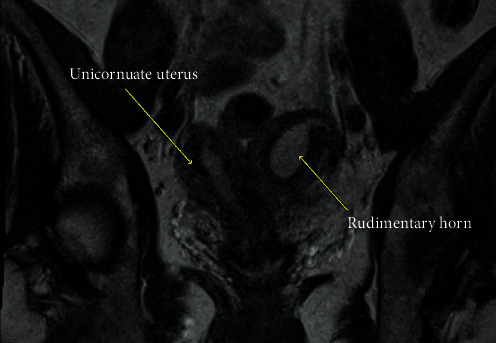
MRI of communicating and noncommunicating horns.
